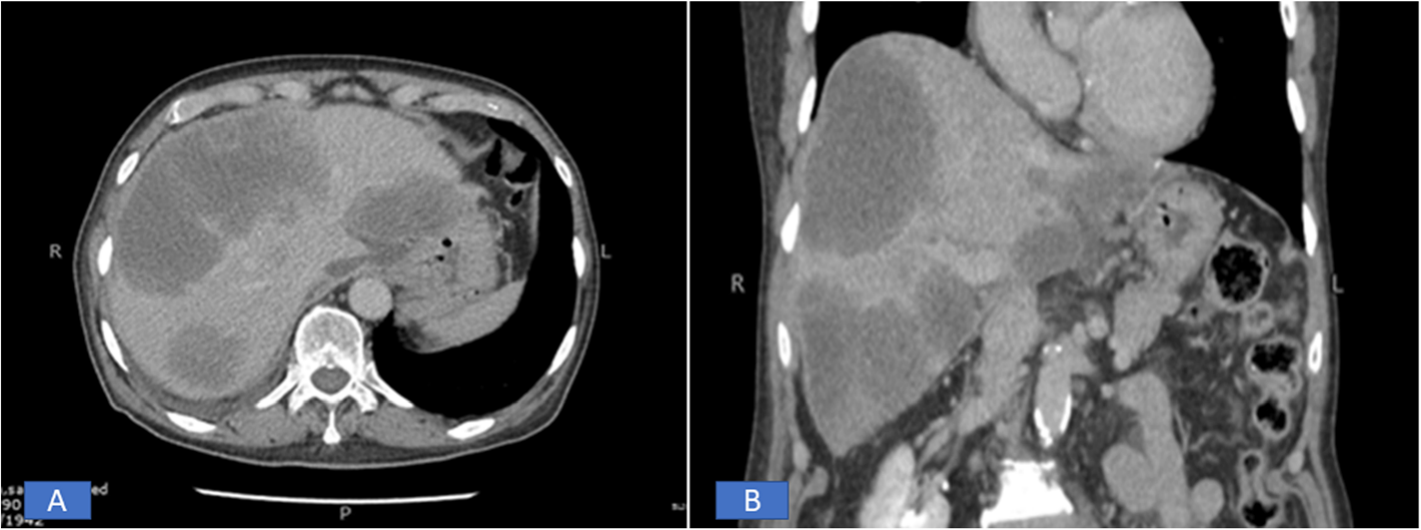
a-b. CT scan images of transverse and coronal section showing multiple large hypodense deposits of varying sizes, in both lobes of the liver. Radiologically, these were interpreted as most likely representing metastatic deposits
